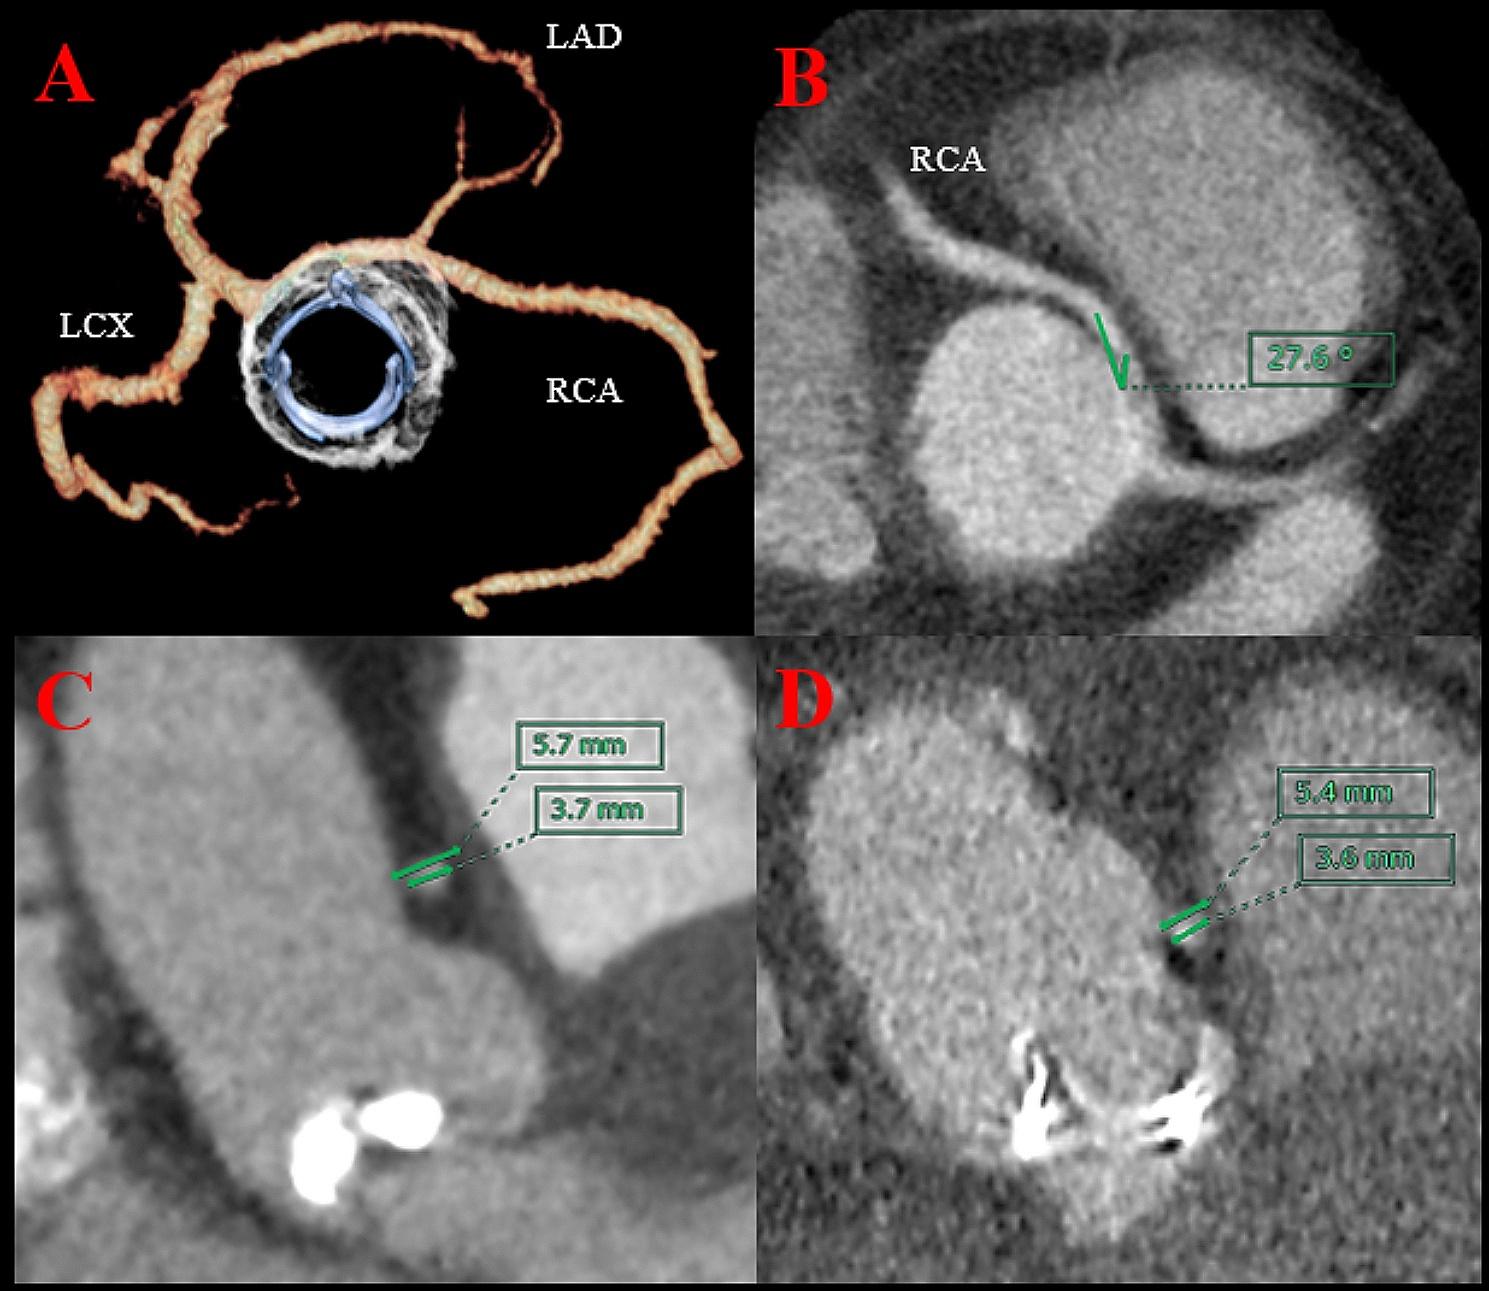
Postoperative cardiac computed tomography. (A) After enlargement, the prosthetic valve was placed without compression of the AAORCA. (B) A take-off angle of the right coronary artery increased from 19° to 28°. (C) The preoperative diameter of the most narrowed point of the interarterial segment and the right coronary artery (RCA) were 5.7 and 3.7 mm, respectively. (D) The postoperative diameter of the interarterial segment and the RCA were 5.4 and 3.6 mm, respectively, and not significantly smaller
